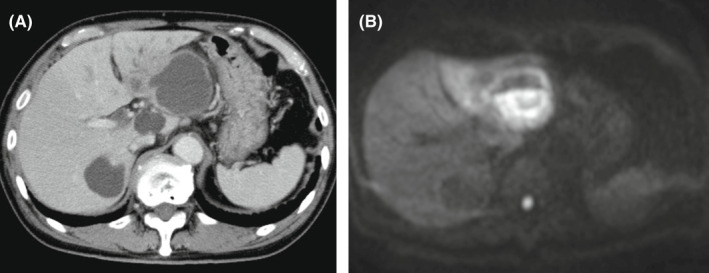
(A) Contrast‐enhanced computed tomography of the abdomen showed no contrast‐enhanced effect on the cyst wall. (B) Diffusion‐weighted magnetic resonance imaging indicated an infected hepatic cyst with high signal intensity
